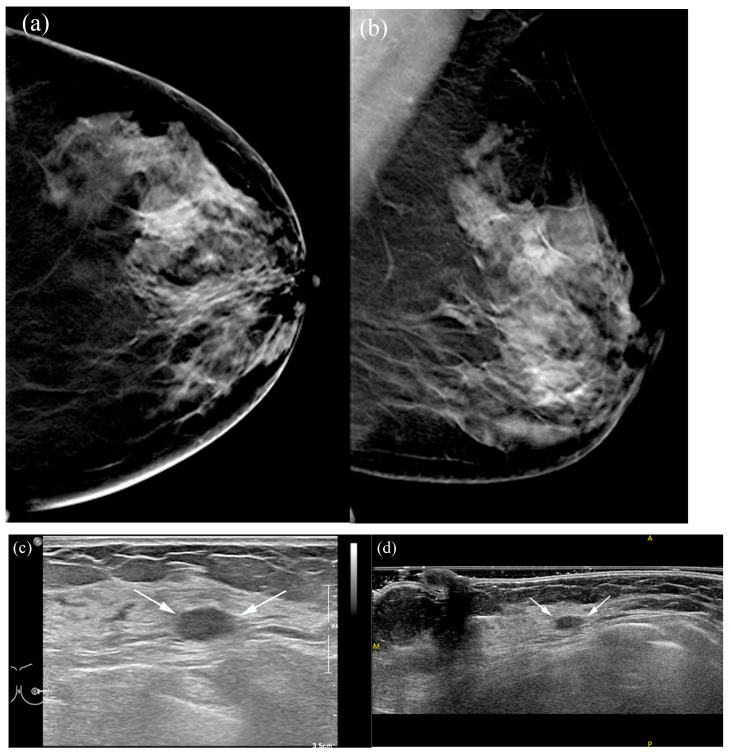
A 50-year-old female patient with left-sided bloody nipple discharge: (a,b) The malignancy is not visualized on digital breast tomosynthesis (DBT). (c) Hand-held ultrasound reveals an indistinct, oval hypoechoic mass (arrows) in the left 3 o’clock area. (d) MammouS-N transverse posteroanterior image shows an indistinct, oval hypoechoic mass (arrows) in the left 3 o’clock area (A, anterior; P, posterior; M, medial). Surgical pathology confirmed microinvasive secretory carcinoma.
